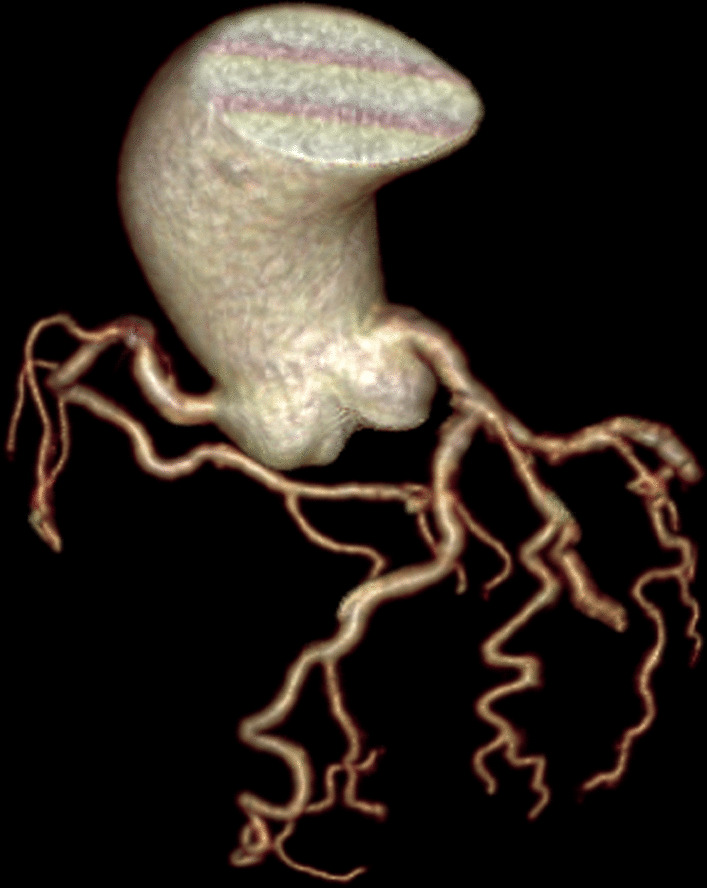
CTA shows tortuous coronary
